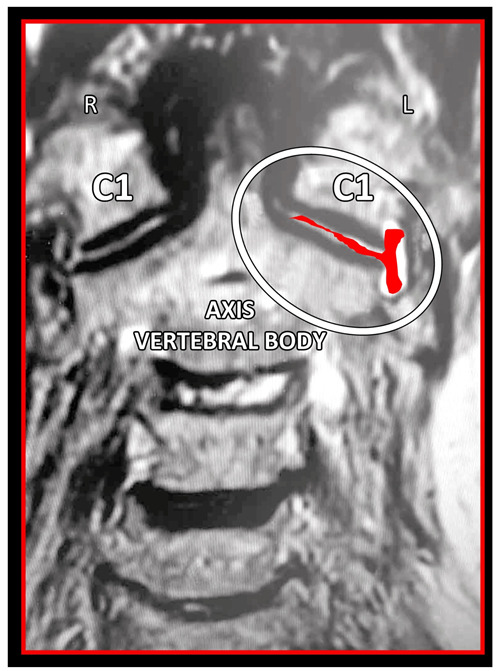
Atlas*-*axis* synovitis. Magnetic resonance imaging showing the left lateral effusion of *atlas*-*axis* intra-joint passive congestion, with increased pain during active axial rotation of the *atlas* on *axis* to either side. C_2_ nerve root involvement with unilateral hemicranial pain.
